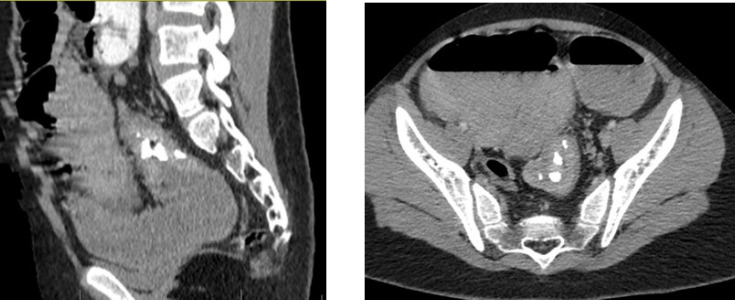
Abdomen and pelvic CT-scan with IV and oral contrast in axial plane (A) and sagittal plane (B) showing hyper-enhancing concentric wall thickening and a mass lesion with dysmorphic calcification
